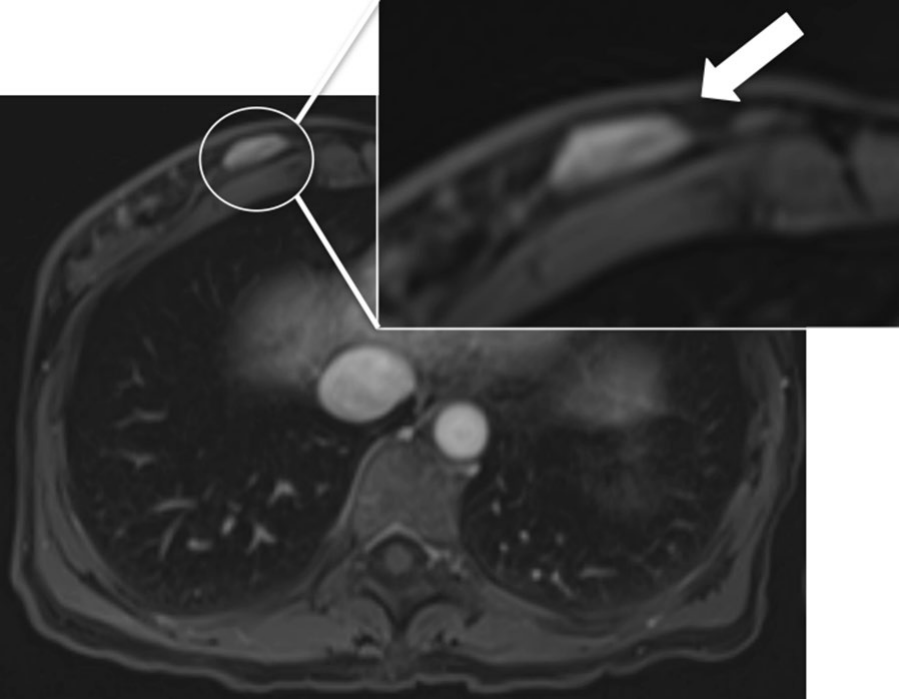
Examples of breast findings by BI-RADS category. A 49-year-woman underwent abdominal MRI for liver evaluation. Three-dimensional dynamic axial volumetric interpolated breath-hold examination image after intravenous power injection of 0.025 mmol/kg of gadoxetic acid (Primovist) at 60 s. Less than 50 % of the breast tissue was visible at abdominal MRI. An oval nodule (*white circle*) was found in the inner quadrant of the right breast (20 mm). The breast finding was first categorized as BI-RADS RM 3. After electronic medical record review this finding was downgraded as BI-RADS RM 2 (known fibrolipoma). In the *upper right corner* is highlighted the breast findings (*white arrow*)
